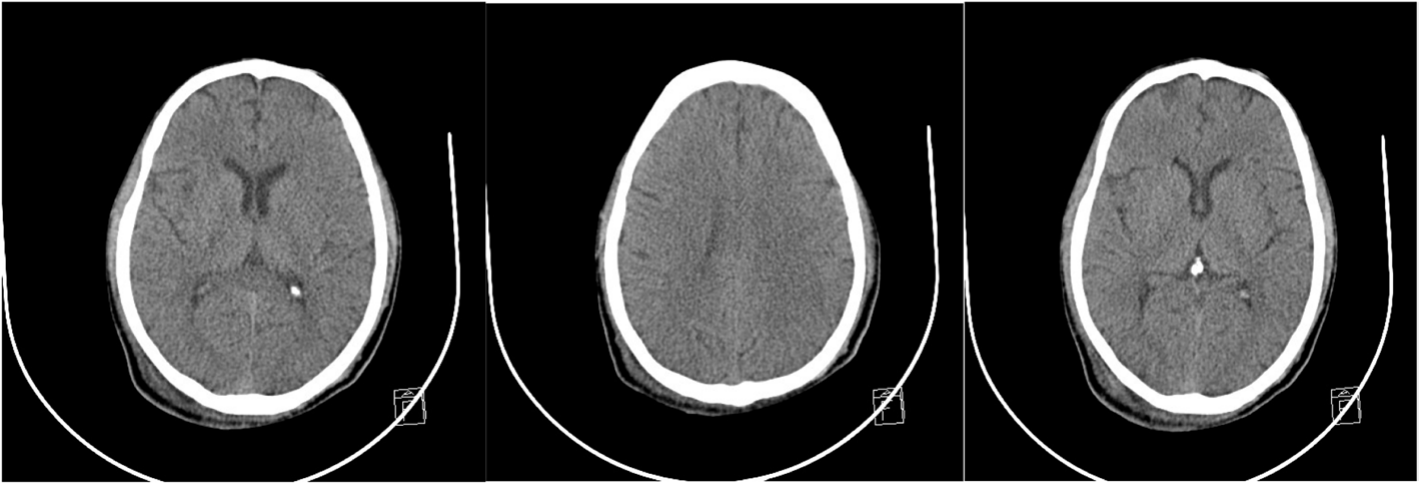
Brain computed tomography scan of the patient
